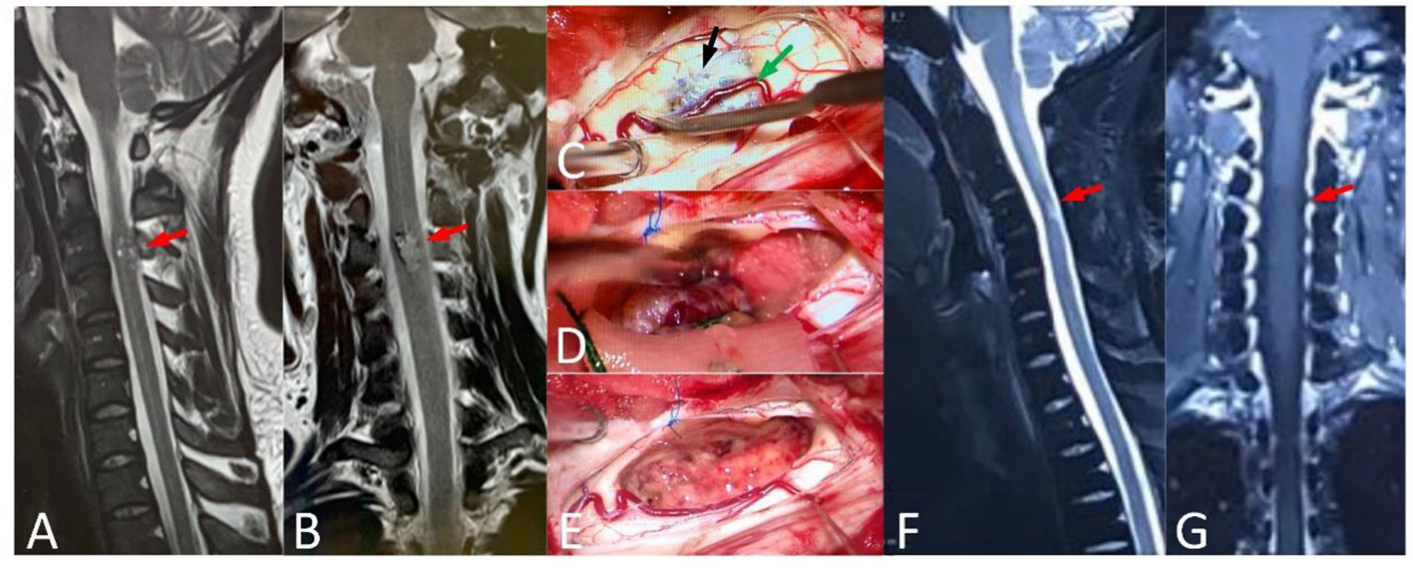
A 37-year-old male, with numbness of limbs and trunk for 5 years, and standing difficulty for 5 days (mALs = 13 points, severe disorder, Type A), was treated by ERS. (A,B) Preoperative spinal MRI examination revealed ISCH in C3 (red arrow). (C–E) The lesion was visible on spinal cord surface (black arrow) and the artery (green arrow) was protected carefully. (F,G) MRI re-examination showed no residual or recurrence in the operative area at 4 years after surgery and the mAS was 7 points at the last follow-up.
